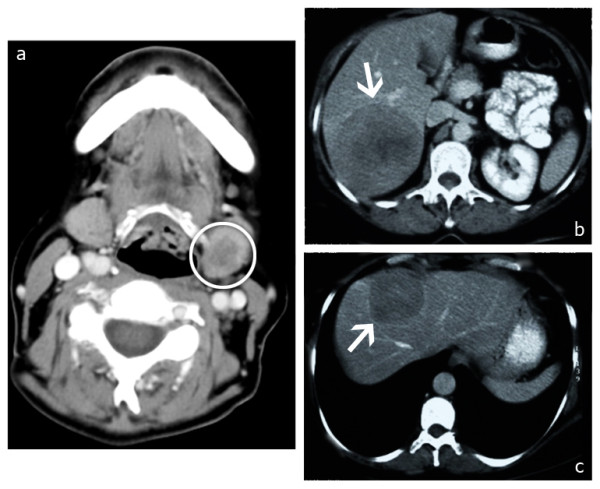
Pre-therapy imaging. (a) The primary tumor. Adenoid cystic carcinoma of the left submandibular salivary gland is indicated by the circle. (b) and (c) Computed tomography (CT) of the liver before chemoembolization showing the two main lesions. (b) A 10 cm lesion involving segments VI and VII is indicated by the arrow. (c) A 4.7 cm lesion involving segments VIII and IV_A _is indicated by the arrow. Three more lesions in segments IV_A_, IV_B_, and II, which measured 1.5 cm, 1 cm, and 1.8 cm, respectively, were identified.
